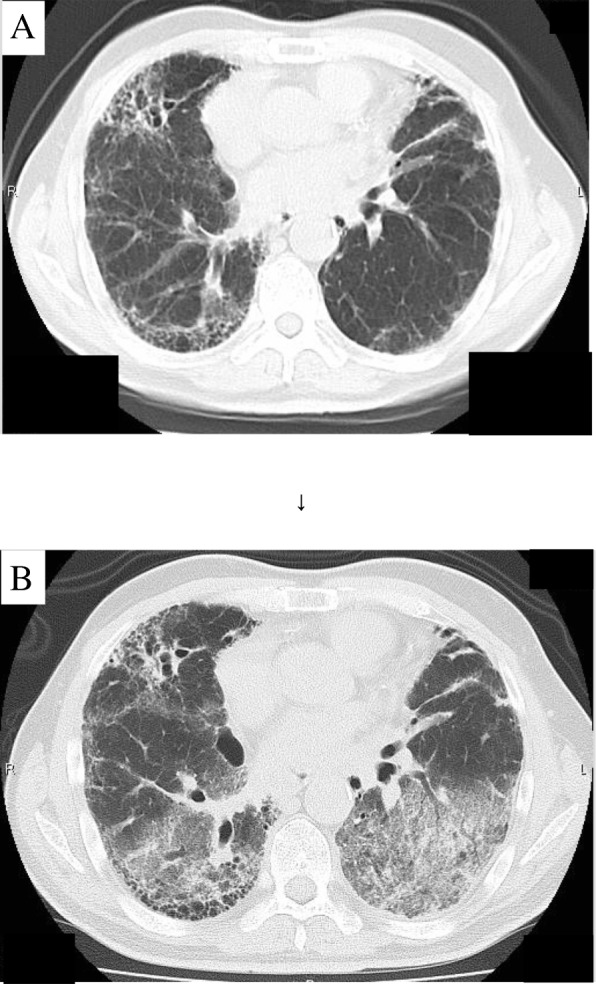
Radiological findings. Chest CT findings for a 69-year-old man. a Chest CT findings at 3 weeks before admission. b Chest CT findings at the time of admission showed new ground-glass opacity findings over a broad range of bilateral lung fields
